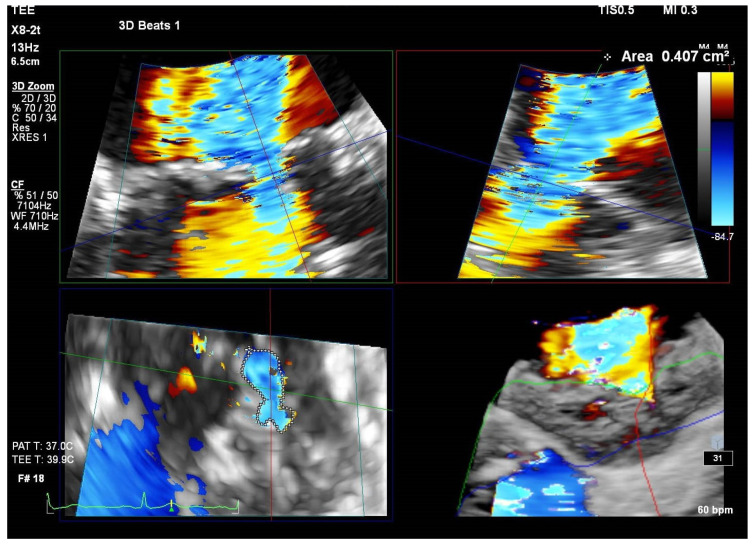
Measurement of vena contracta area by 3D TEE. (3D TEE: three-dimensional transesophageal echocardiography).
